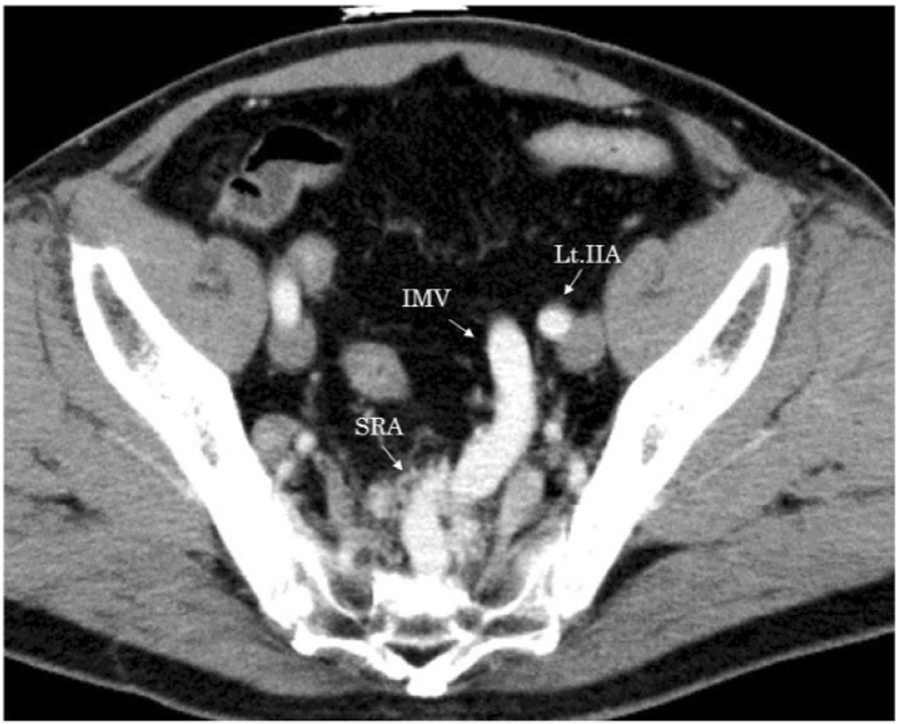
Enhanced computed tomography showed dilation of inferior mesenteric vein (IMV) and superior rectal artery (SRA) was noted in arterial phase. Lt IIA: left internal iliac artery
